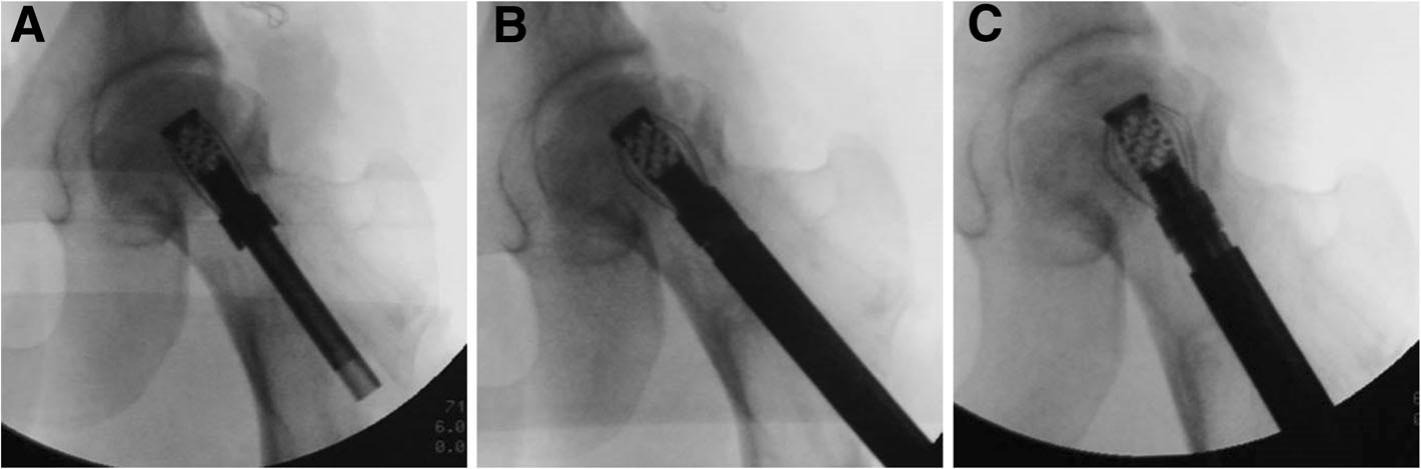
Intraoperative radiography for evaluation of surgical procedure. a Insertion of the lantern-shaped screw. b The lantern-shaped screw was being unfolded. c The lantern-shaped screw was the achievement of surface at surface support for the subchondral bone of femoral head
